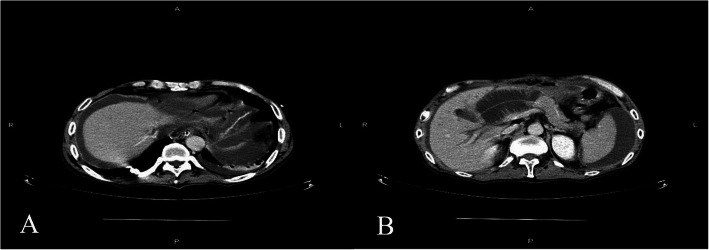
On POD 10, CT showed (a) bilateral subphrenic and intraperitoneal free gas and abdominal pelvic effusion; (b) dilatation and effusion of upper abdominal intestine
